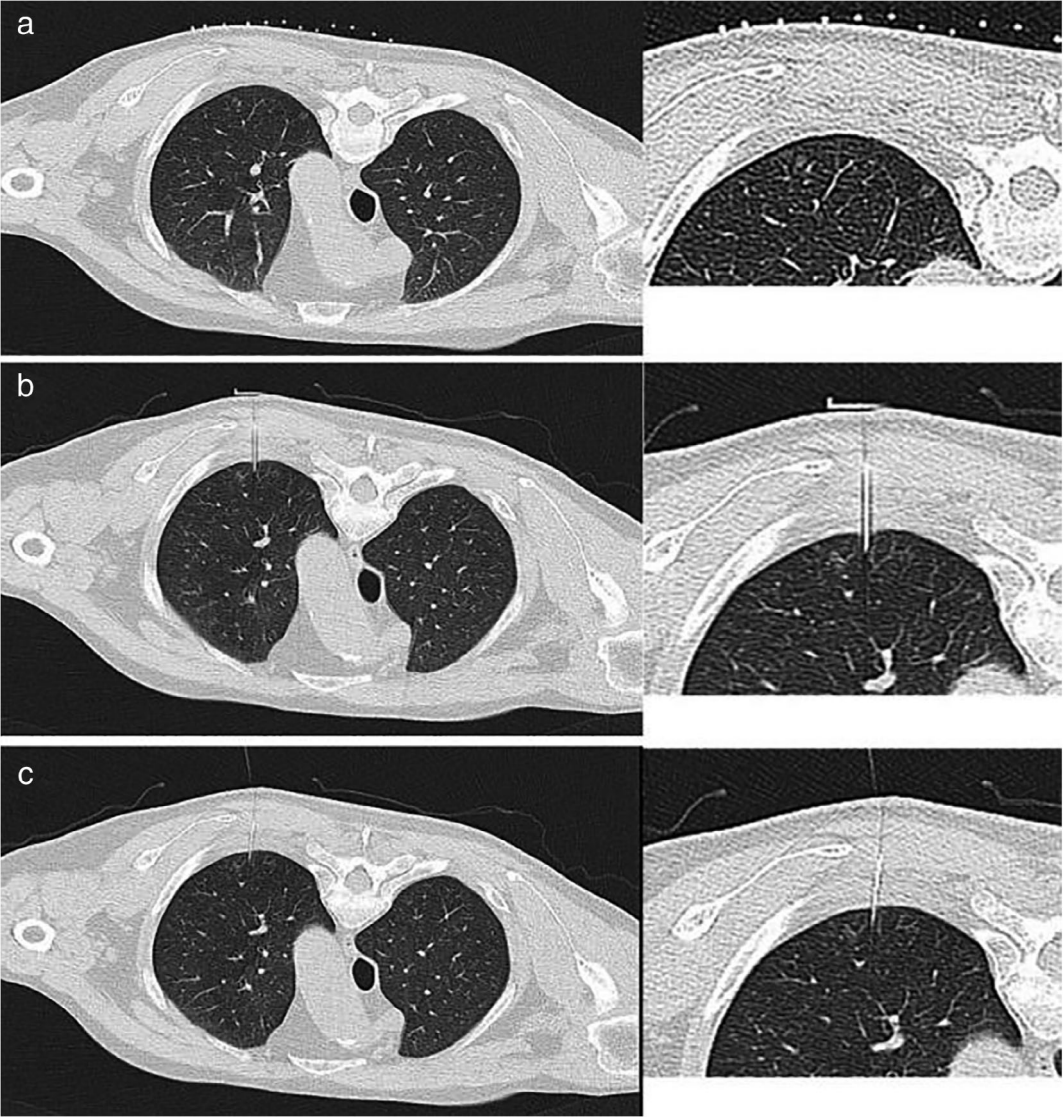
The positioning process of hookwire with CT guided. CT scan chose puncture plane before operation (a), CT scan determines the angle and depth of insertion during operation (b), and CT scan confirmed that the hookwire was located in the lung tissue around the lesion after operation (c).
